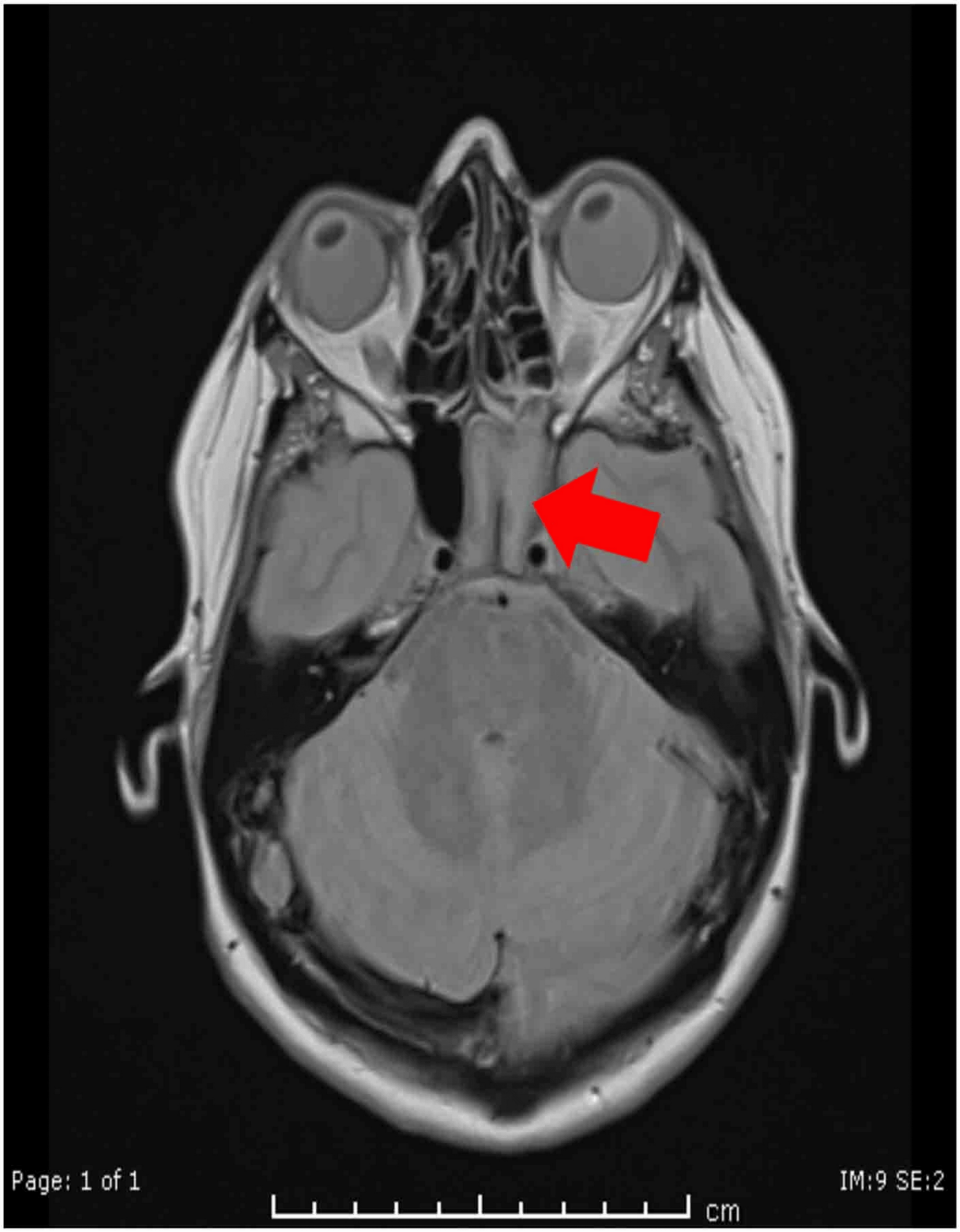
CT of paranasal sinuses of case 2. Computed tomography of the paranasal sinuses demonstrated soft tissue opacification (red arrow) in the sphenoid sinus. IM: inferior meatus; SE: sphenoethmoidal recess.
